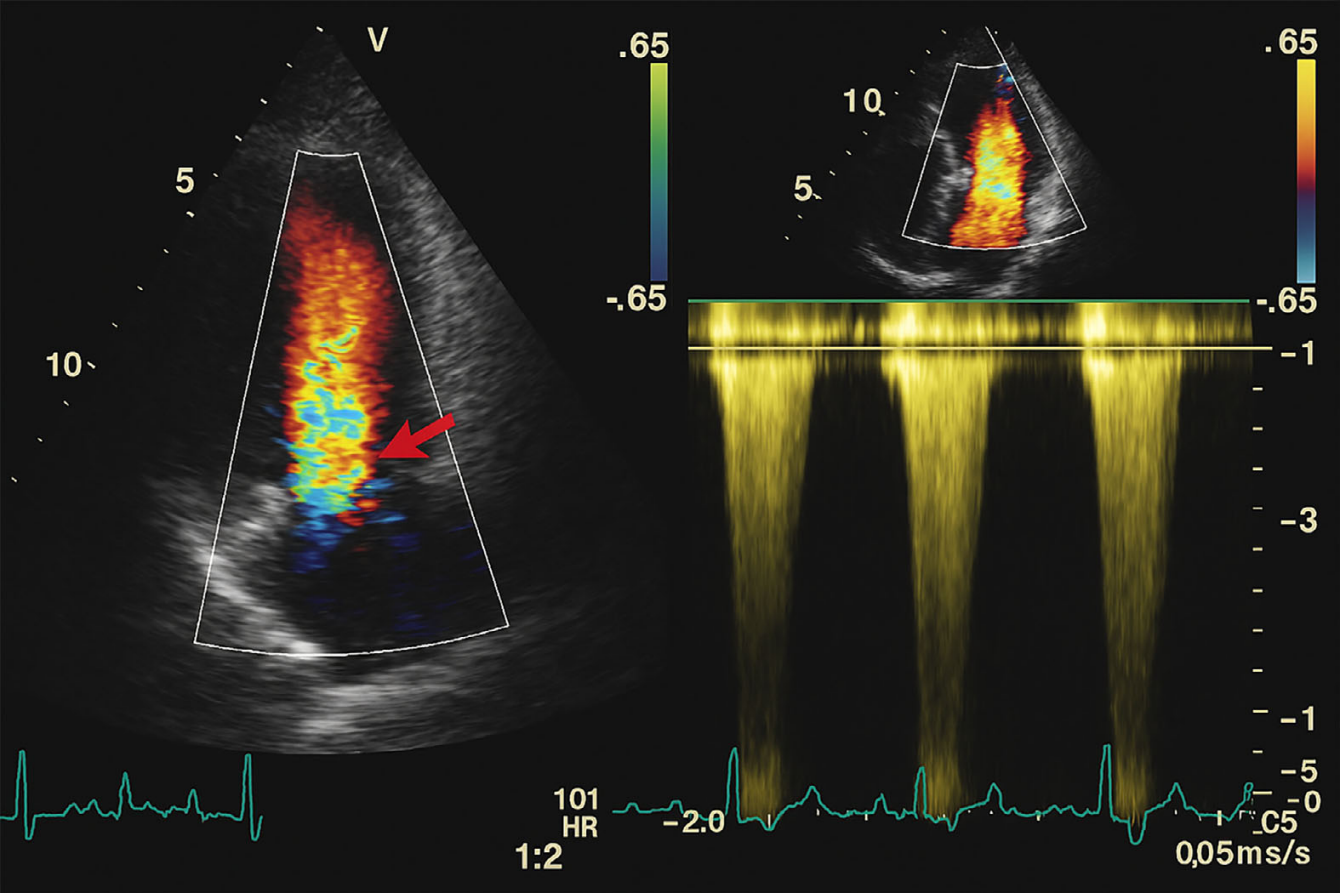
Doppler echocardiographic image demonstrating dynamic left ventricular outflow tract (LVOT) obstruction highlighting a turbulent jet (red arrow) consistent with systolic anterior motion (SAM) of the mitral valve and LVOT obstruction.
